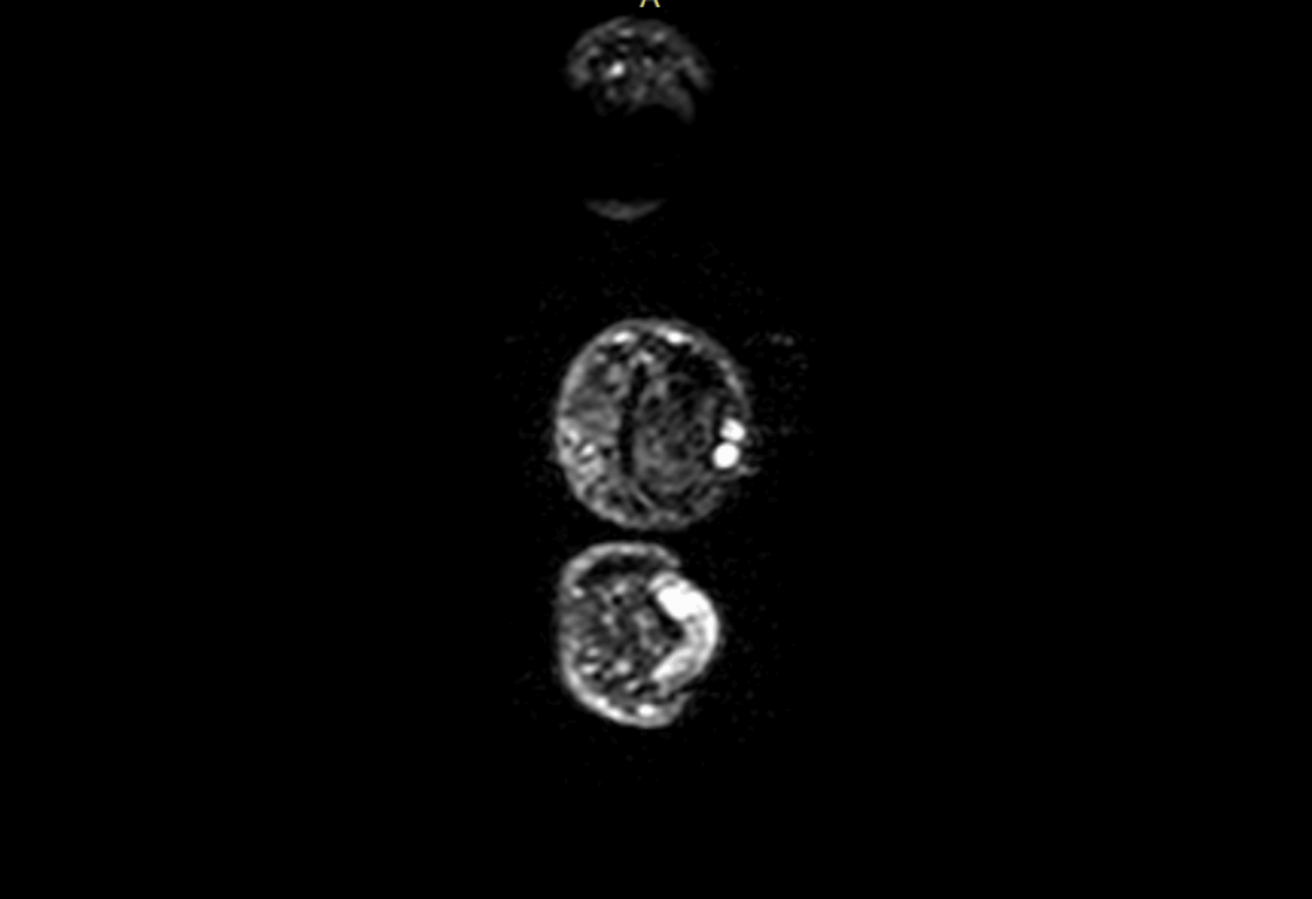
Axial MRI image showing a glomus tumor.
